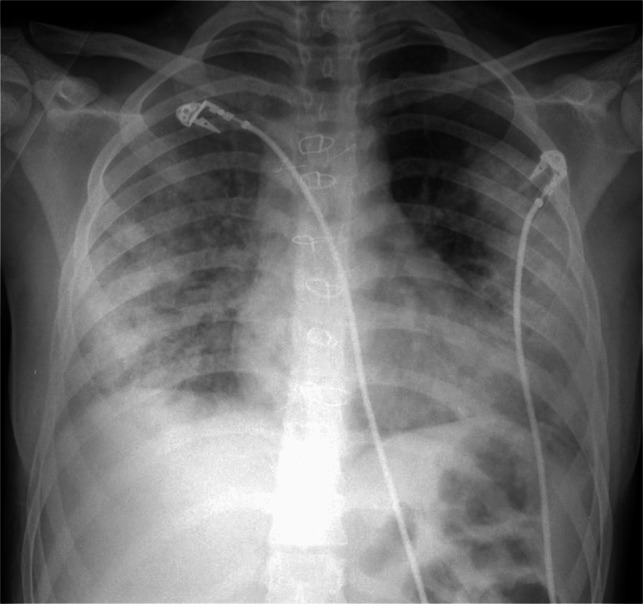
A 17-year-old female with history of cystic fibrosis and lung transplant receiving doxycycline. Anteroposterior chest radiograph showing extensive bilateral peripheral predominant consolidation typical of chronic eosinophilic pneumonia. Temporal relationship to doxycycline administration and response to steroid therapy supported a diagnosis of doxycycline-induced lung disease
